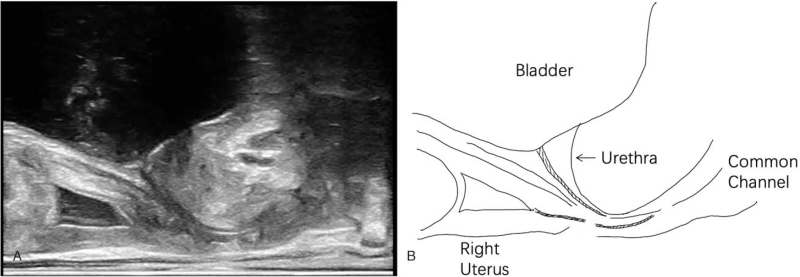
Transrectal biplanar high-frequency ultrasonography showing the urethra was bent at 90°, and the distal vagina was not identified.
